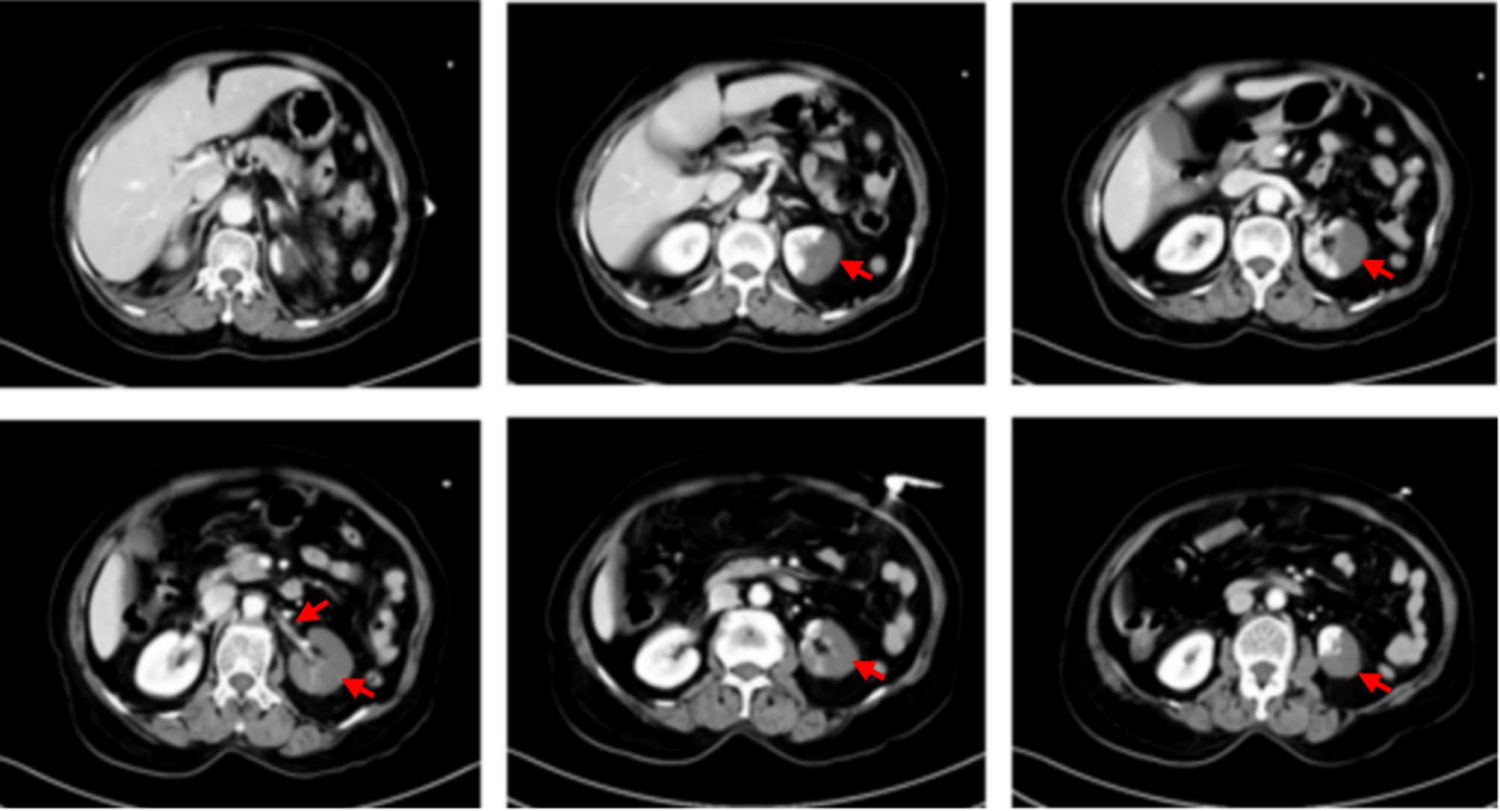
Contrast-enhanced CT of the abdomen and pelvis. The main trunk of the left renal artery appears narrower than the right, and a large region of the left kidney shows no contrast enhancement, indicating infarction (highlighted by the arrow)
